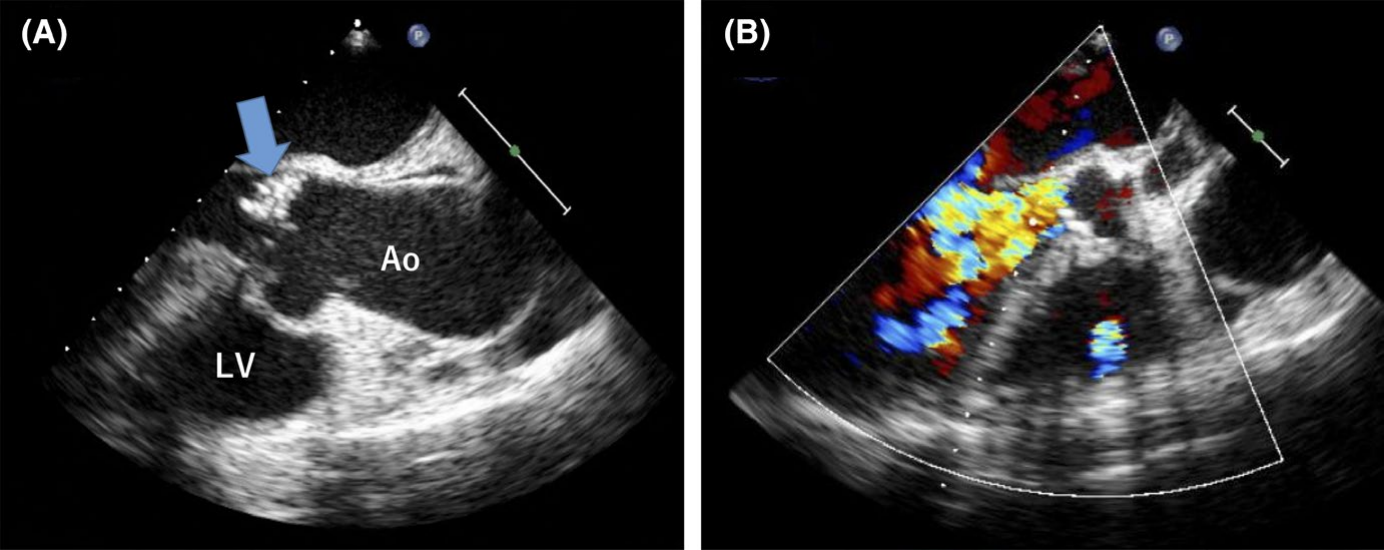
A, Transesophageal echocardiography on day 36 showing a 1.5‐cm motile vegetation (blue arrow) on the noncoronary cusp of the aortic valve. B, Severe atrial regurgitation
